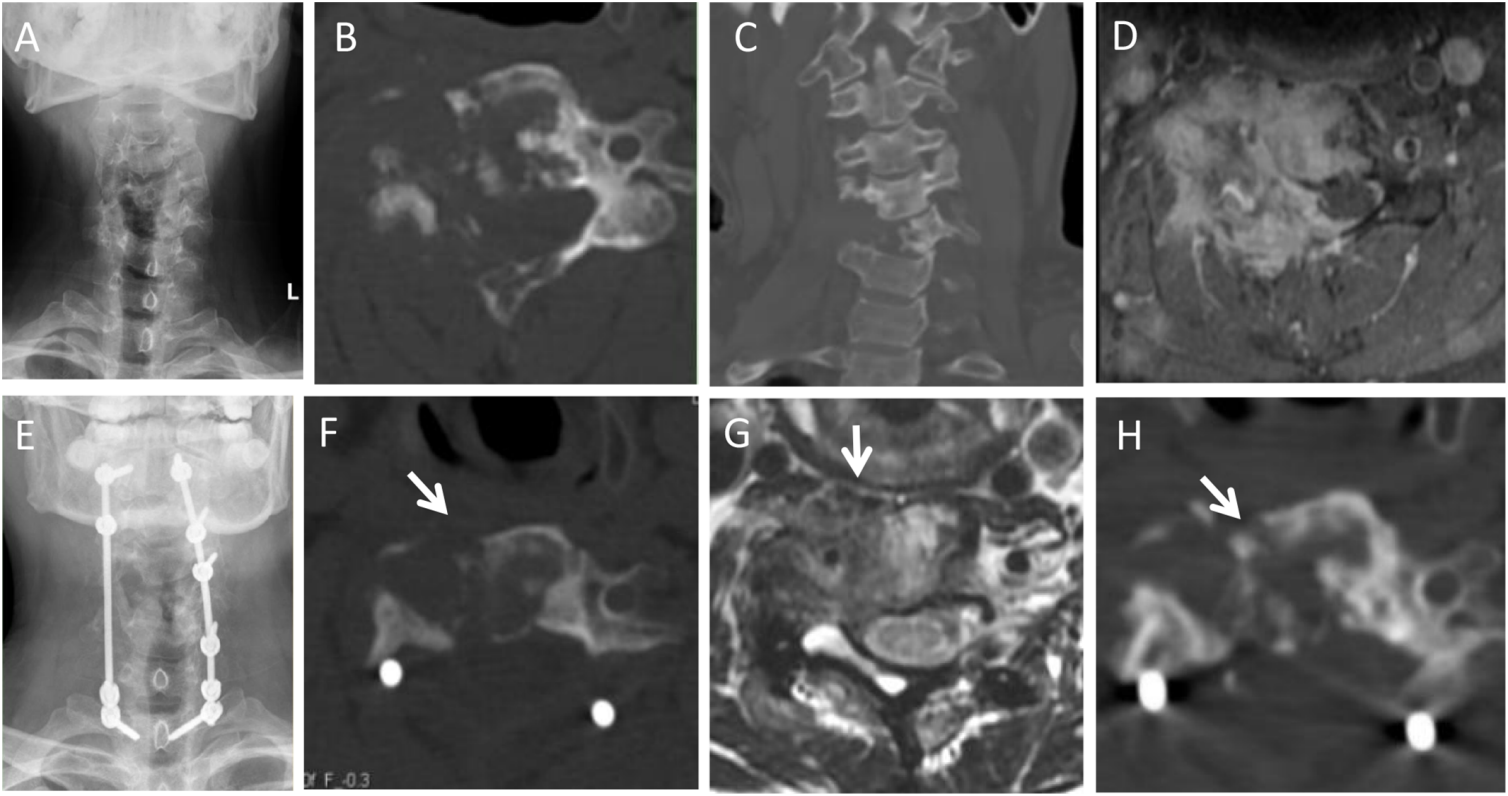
A 28-year-old male with neck pain for 12 months, worsened and accompanied with radiating pain in right upper extremity, shoulder and back, numbness in right hand for six months. Preoperative imaging examination showed a irregular and osteolytic lesion located in C4-6 with scoliosis. GTR was panned, but he underwent STR for strong adhesion between tumor and dural sac, which was revealed during the operation. CT and MRI scans revealed soft tissue mass and osteolytic lesion of local progression at 3-month follow-up after surgery (arrows in F,G). He underwent radiotherapy. The lesion was stable with partial osteogenesis in 24-month follow-up after radiotherapy with new bone (arrow in H). (A): Preoperative X-ray; (B,C): Preoperative axial and coronal CT scans; (D): T1-weighted axial contrast enhanced MRI scan; (E): Postoperative X-ray; (F): Axial CT scan at 3-month follow-up after surgery; (G): T2-weighted axial MRI scan in 3rd month after surgery; (H): Axial CT scan at 24-month follow-up after radiotherapy.
